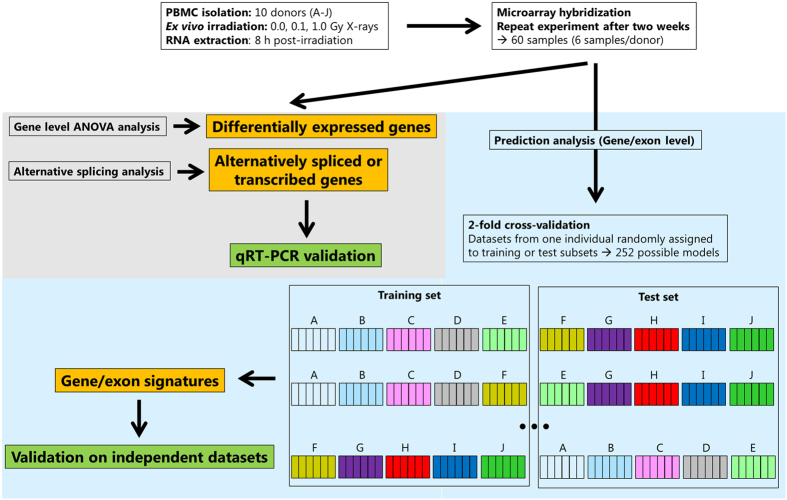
Outline of the strategy to identify gene and exon signatures suitable for prediction of exposure to doses of 0.1 and 1.0 Gy of X-rays. Isolated PBMCs from 10 healthy donors were irradiated and RNA was extracted 8 h after irradiation. RNA was hybridised onto Affymetrix Human Gene 1.0 ST arrays and differentially expressed and alternatively spliced genes were identified and validated using qRT-PCR. Three classification algorithms were employed to identify predictive gene and exon signatures and their predictive performance was tested using 2-fold cross-validation (boxes labeled with letters A–J represent all the samples from each out of ten donors (0.0, 0.1 and 1.0 Gy, in duplicates). The best performing gene signature as identified by the RF model was further evaluated on an independent, publicly available dataset.
